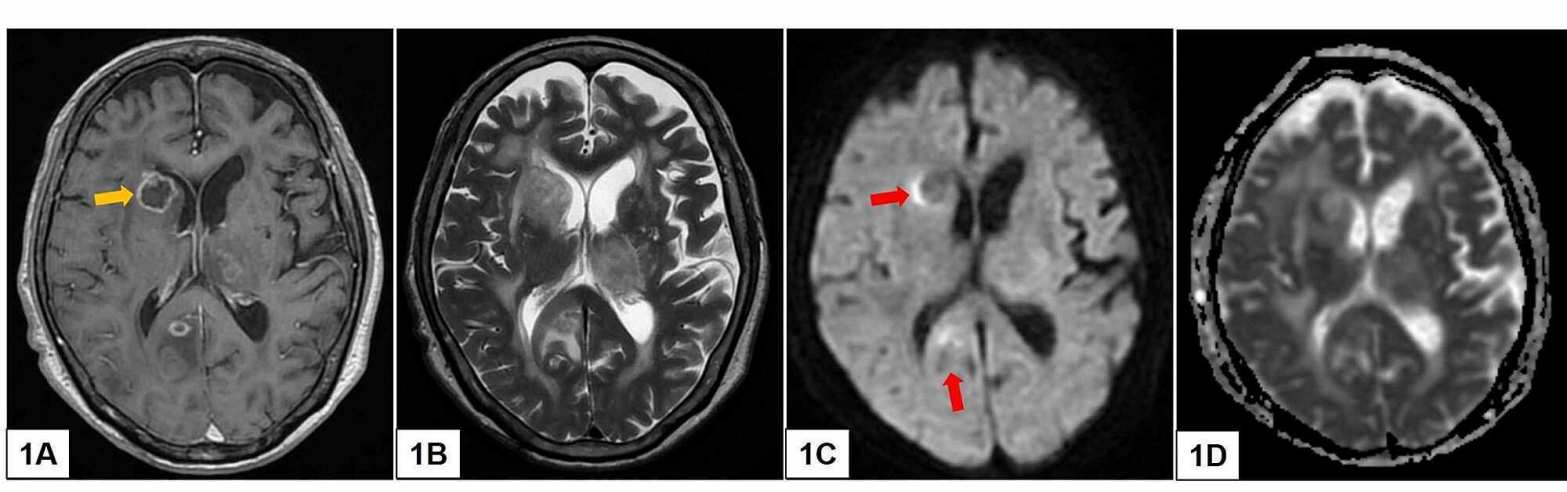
Representative MRI images in axial view in T1-weighted, post-contrast (A), T2-weighted (B), DWI (C), and its corresponding ADC map (D). Here, the imaging shows multiple, ring-enhancing intraparenchymal lesions of different sizes (A). Some of the lesions are associated with vasogenic edema (B). There is also evidence of restricted diffusion (red arrows) in (C) and (D). Of note, the ring-enhancing lesion adjacent to the right frontal ventricular horn (yellow arrow) is selected for the subsequent stereotactic biopsy. DWI, diffusion-weighted imaging; ADC, apparent diffusion coefficient
